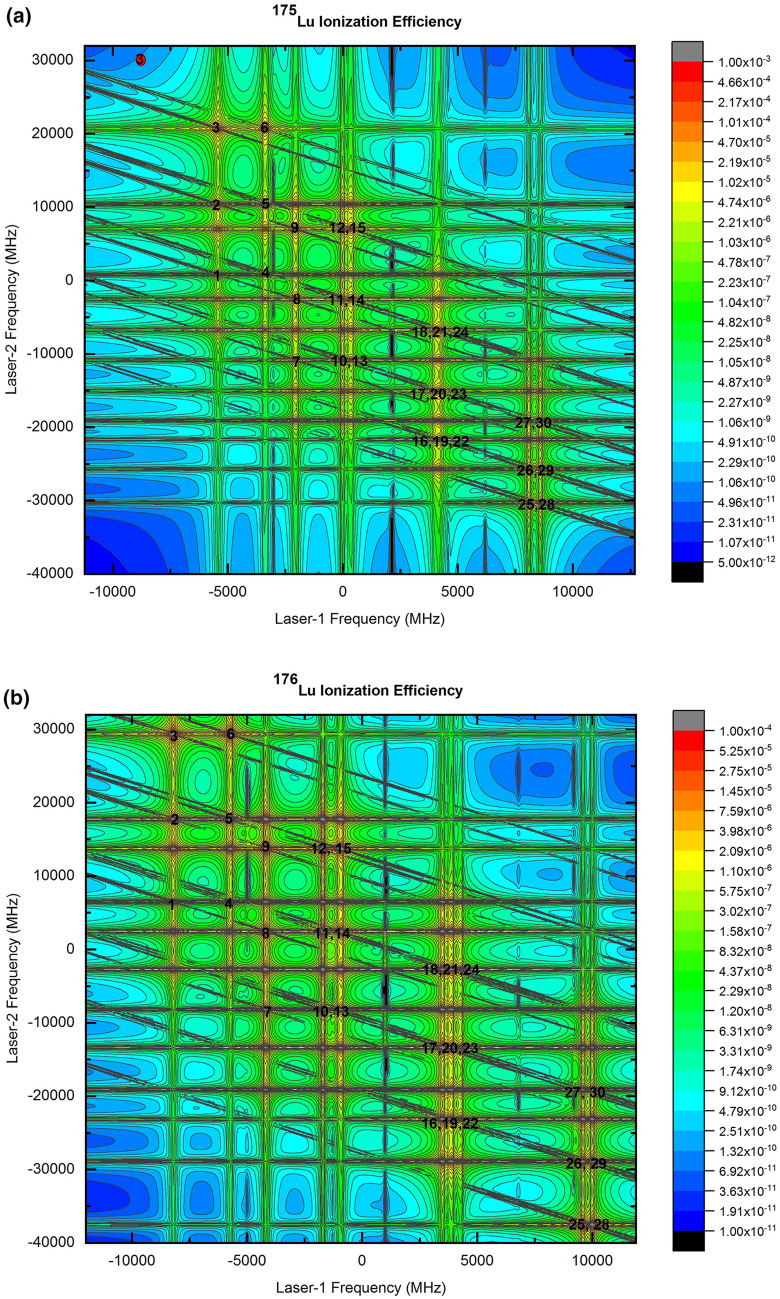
Two dimensional contour of the ionization efficiency of (a) ^175^Lu and (b) and ^176^Lu for the Doppler free condition and for a laser peak power density of 10 W/cm^2^ and a bandwidth of 10 MHz for the excitation lasers. The resonance frequency positions of the hyperfine excitation pathways are numbered as per the Table 2. The resonance frequency position of the 17/2–17/2–19/2 of the ^176^Lu is shown as “filled circle” in (a).
